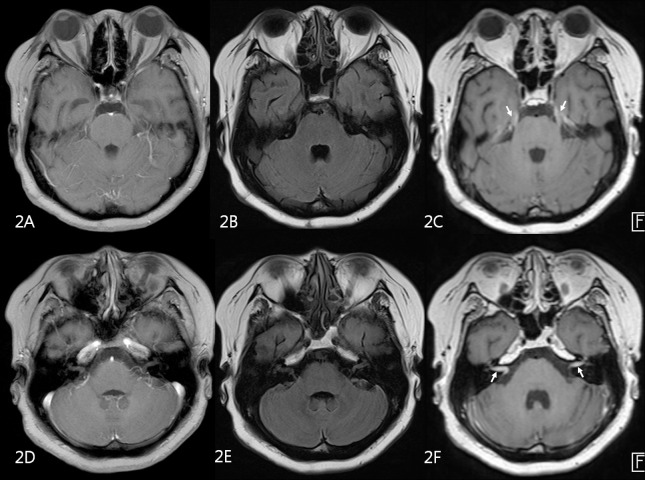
MR images of 58 year-old male patient with advanced gastric cancer. 2D contrast-enhanced T1-weighted GRE (A,D) and 2D FLAIR (B,E) were negatively interpreted by both faculty and trainee raters. On 3D contrast-enhanced T1-SPACE (C,F), leptomeningeal enhancement along trigeminal nerves (arrows on C) and internal auditory canals (arrows on F) was seen.
